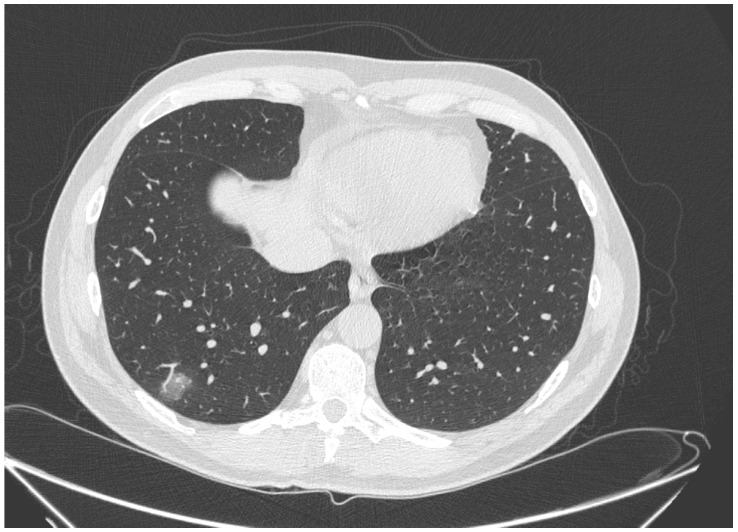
CT examination of a patient with a positive RT-PCR test for COVID-19 infection. The same CT shows a single localization of GGO in the right lower lobe with a rounded morphology.
